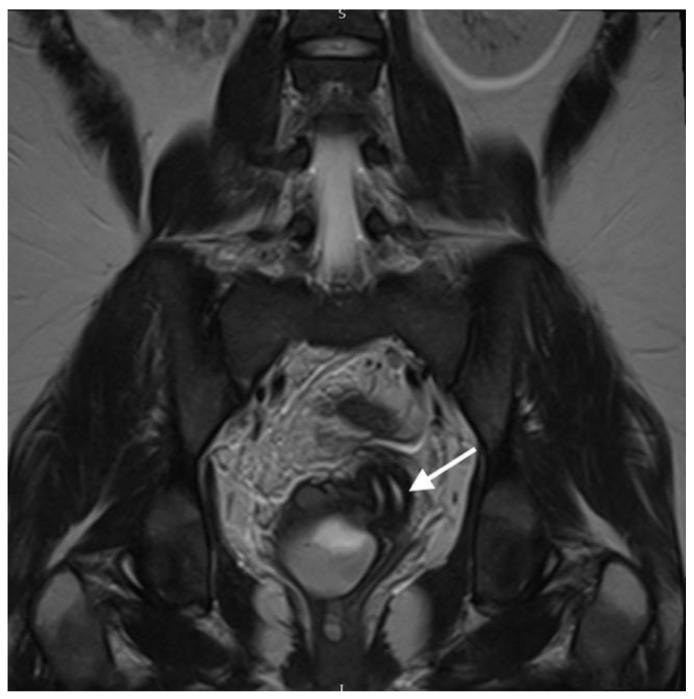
Coronal T2-weighted MR image shows 2 cervical canals (White arrow indicates the two cervical canals. The right-sided cervix leads to the obstructed haematopyocolpos). OHVIRA syndrome, also known as Herlyn–Werner–Wunderlich syndrome, is a rare uterine and renal congenital abnormality which is characterized by an obstructed hemivagina and ipsilateral renal agenesis [2]. The exact prevalence of the syndrome has not been yet estimated, but it has been reported that it accounts for 0.16–10% of Mullerian abnormalities, the incidence of which is 2–3% of the population [3]. Assessment via pelvic ultrasound in combination with MRI helps to establish an accurate clinical diagnosis [4]. In the majority of cases, pubertal women are more likely to be identified with the syndrome, since the onset of menstrual periods might lead to hematometra and/or delayed menarche. However, in cases of partial obstruction, the diagnosis can be elusive, often taking years to be established, leading, at times, to women presenting in adulthood with symptoms resembling pelvic infection and endometriosis [2]. Septectomy remains the treatment of choice and should be performed in all affected patients. Knowledge of this rare condition and maintaining high levels of suspicion is critical in these cases [5,6].
